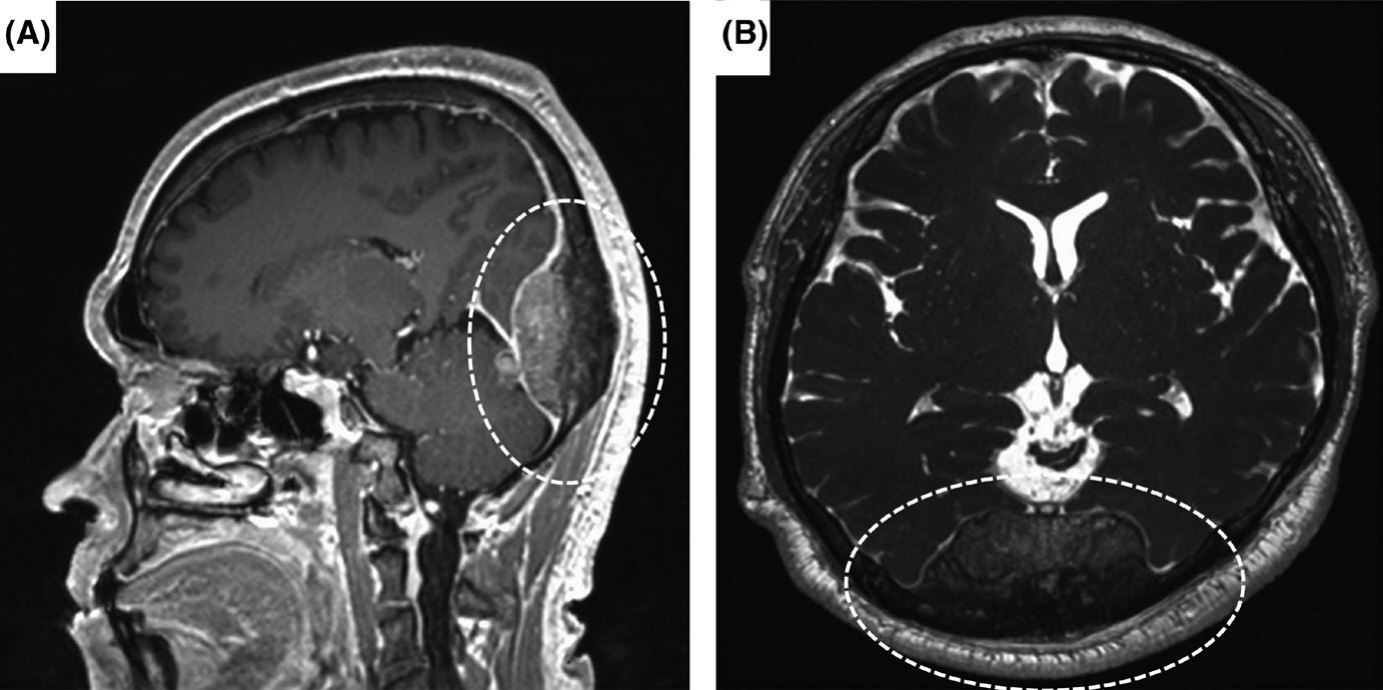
Cranial magnetic resonance imaging with contrast enhancement. (A) Sagittal T1‐weighted magnetization‐prepared rapid gradient echo image. (B) Axial T2‐weighted constructive interference in a steady‐state image. Magnetic resonance images showing a well‐defined mass in the mid‐occipital region from the occipital bone to the epidural space, with slightly low intensity on T1‐weighted images (A), slightly heterogeneous and low intensity at the margins, and mildly high intensity at the center on T2‐weighted images (B). The lesion in the bone is low intensity in both T1‐ and T2‐weighted images
